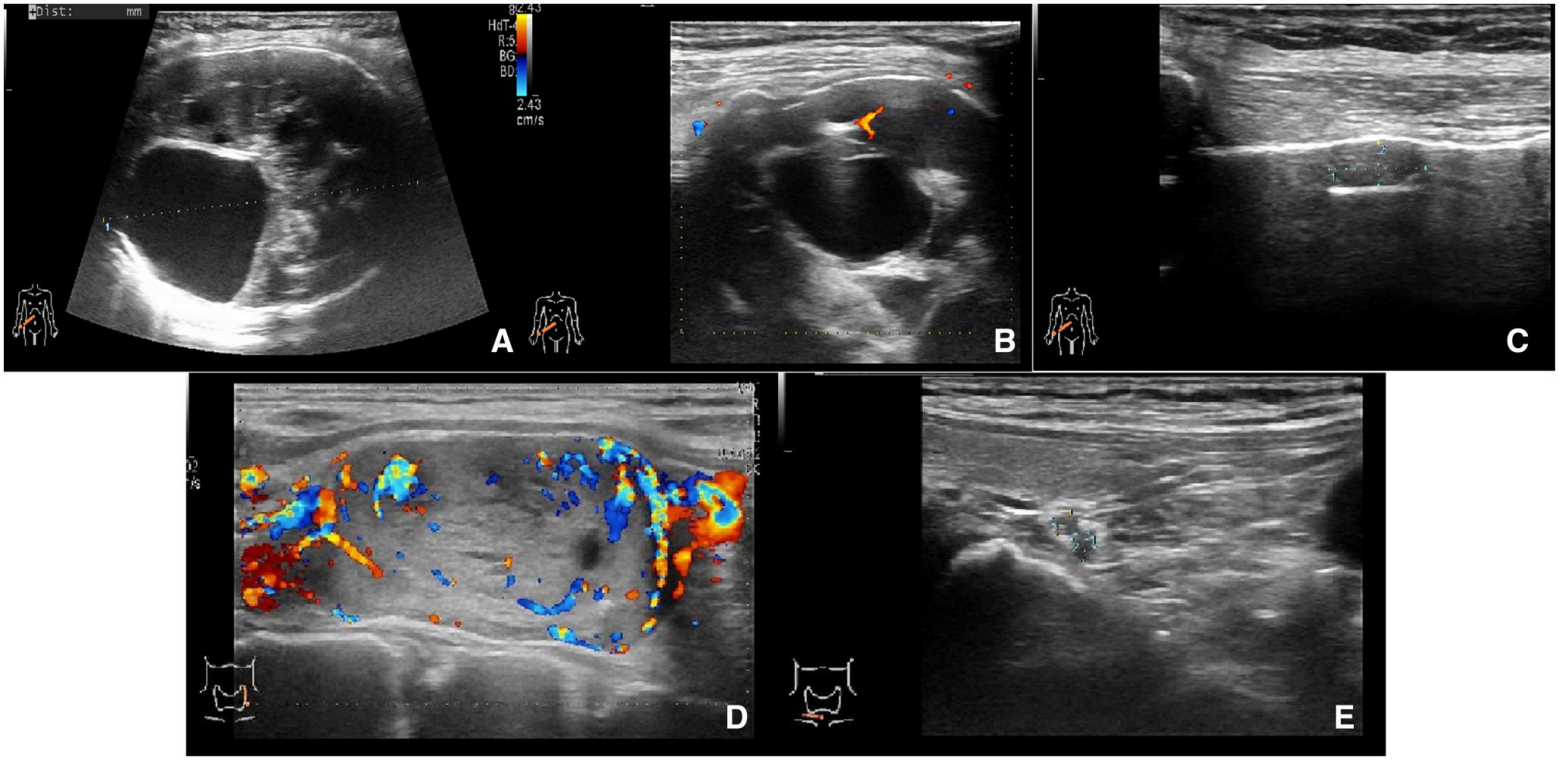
(A-C) Ultrasound shows heterogeneous hypoechoic masses in the tenth rib of the right chest wall, and the colour doppler flow image shows punctate blood flow signal inside the solid part. (D, E) Parathyroid ultrasonography shows heterogeneous/hypoechoic nodules in the left upper and right lower parathyroid glands.
